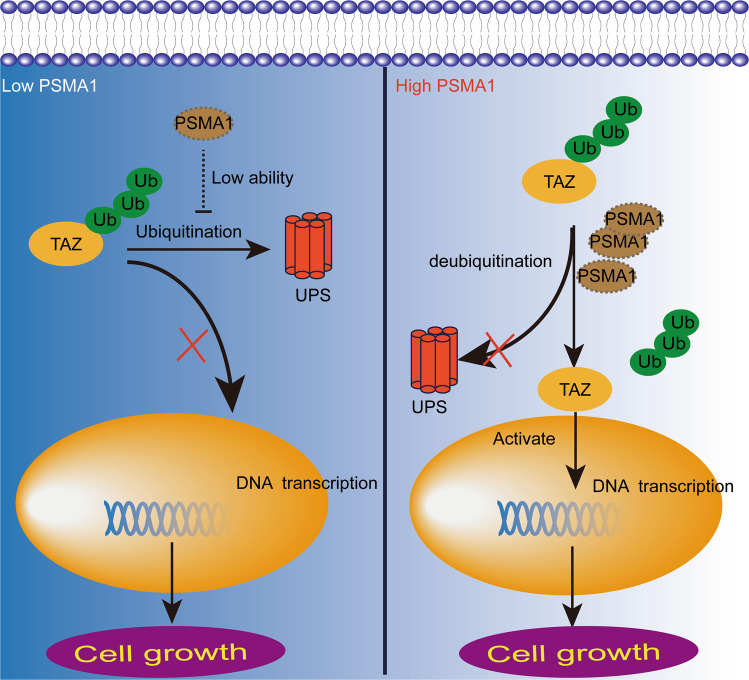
Scheme for the regulatory mechanism of PSMA1 on TAZ.
